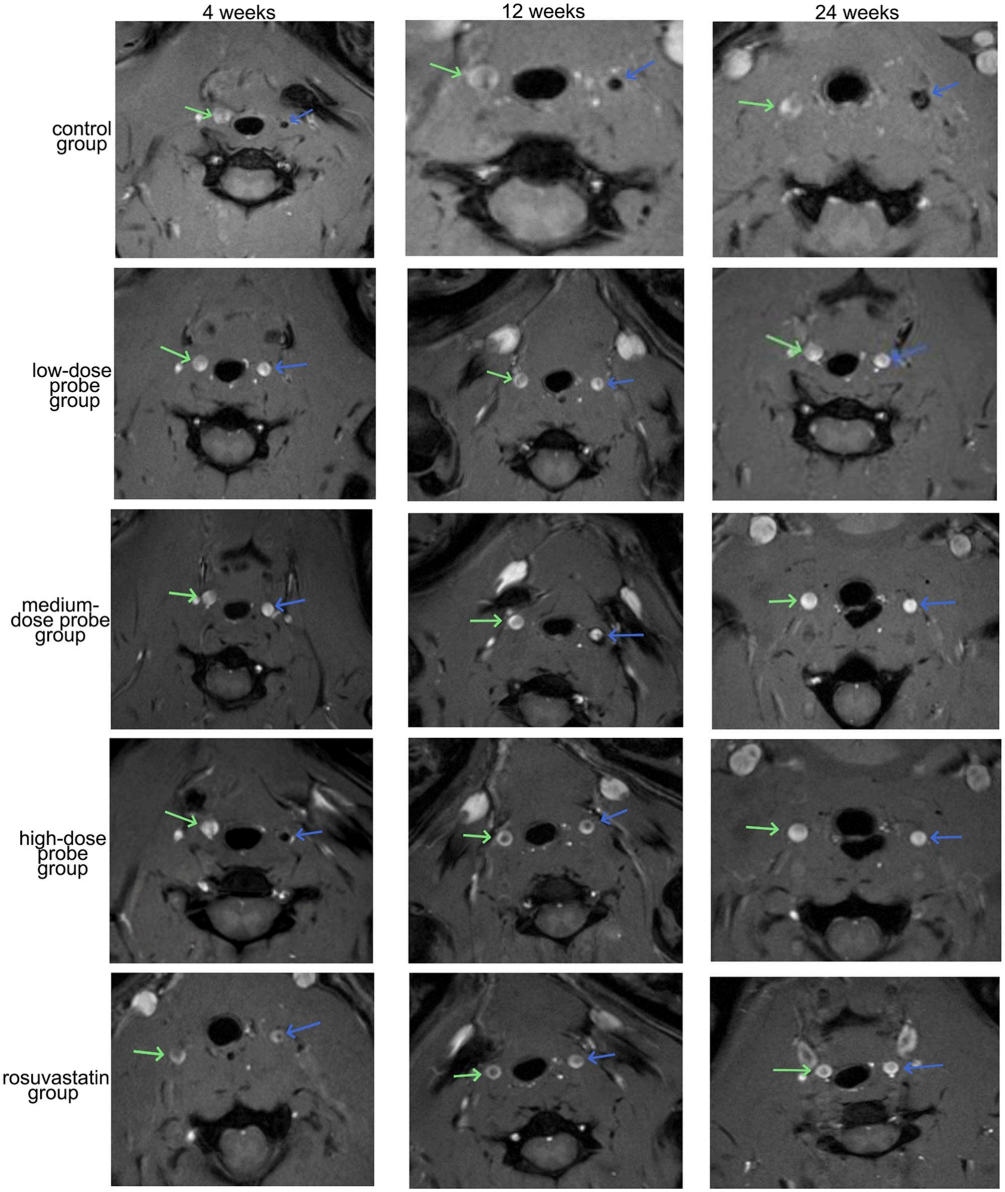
Representative images of MRI detection of aneurysm angiography. The blue arrow indicates the left carotid artery, and the green arrow indicates the right carotid artery.
